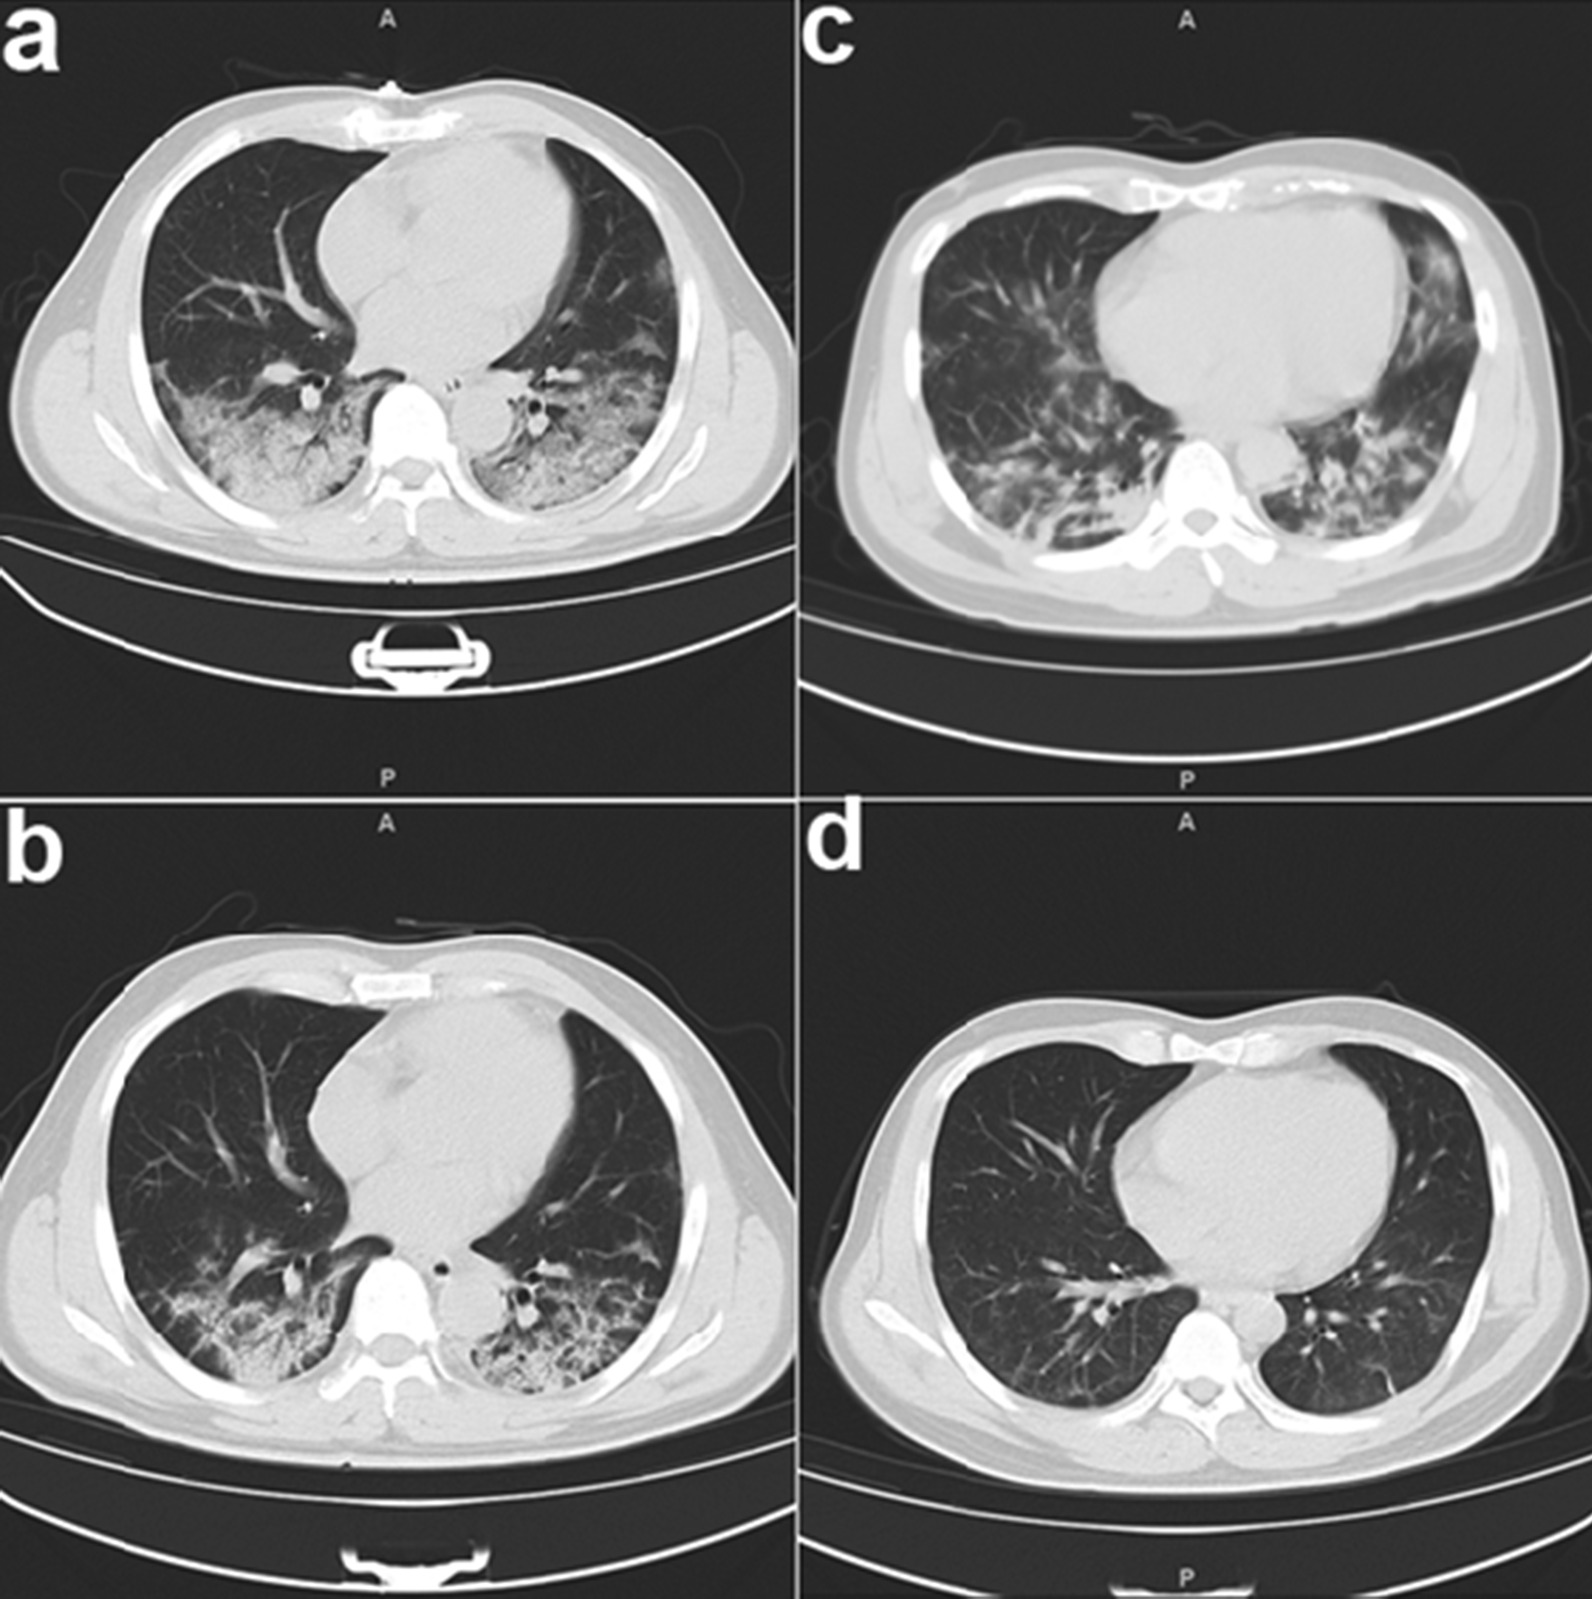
CT scans of the lungs of two representative patients with severe COVID-19 before treatment (A and C) and after treatment (B and D). Note the reduced lesion size and inflammation after treatment
